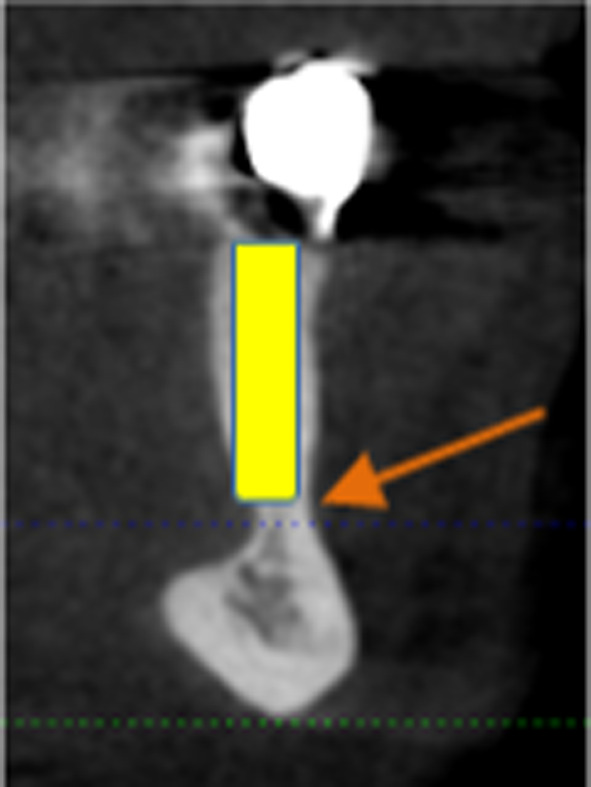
CBCT with virtually planned implant planning in region 35 showing a funnel-shaped contour of the jaw and a pronounced retraction of the jaw contour from the lingual side. The funnel-shaped jaw contour is invisible in the panoramic radiography. Perforation of the jaw can be prevented through CBCT images
